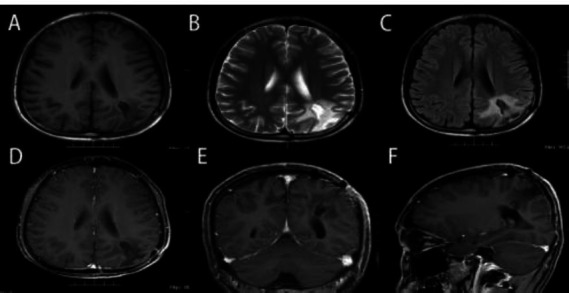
(A-F) After surgery, the patient underwent radiation therapy and chemotherapy. After 18 months, an MRI examination was performed, and no residual tumor or recurrence was found.
